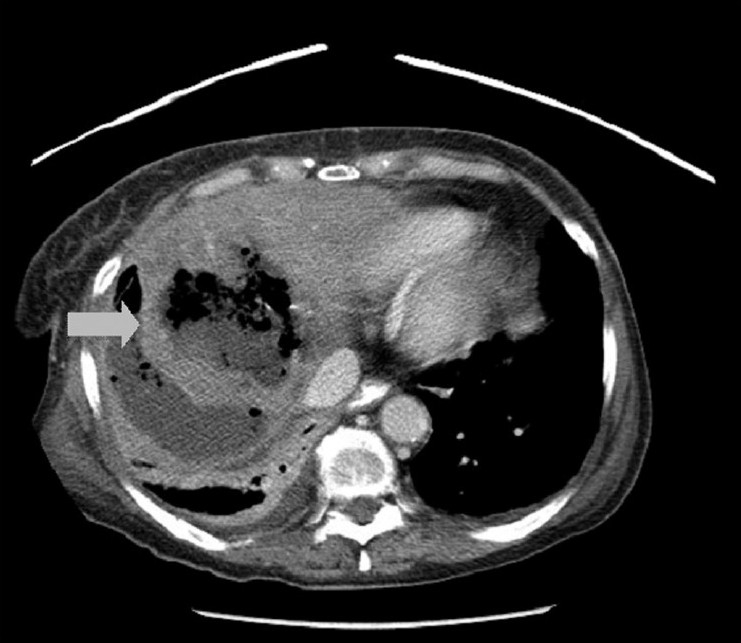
CT scan of the abdomen on post-operative day 9 demonstrating intra-hepatic abscess
